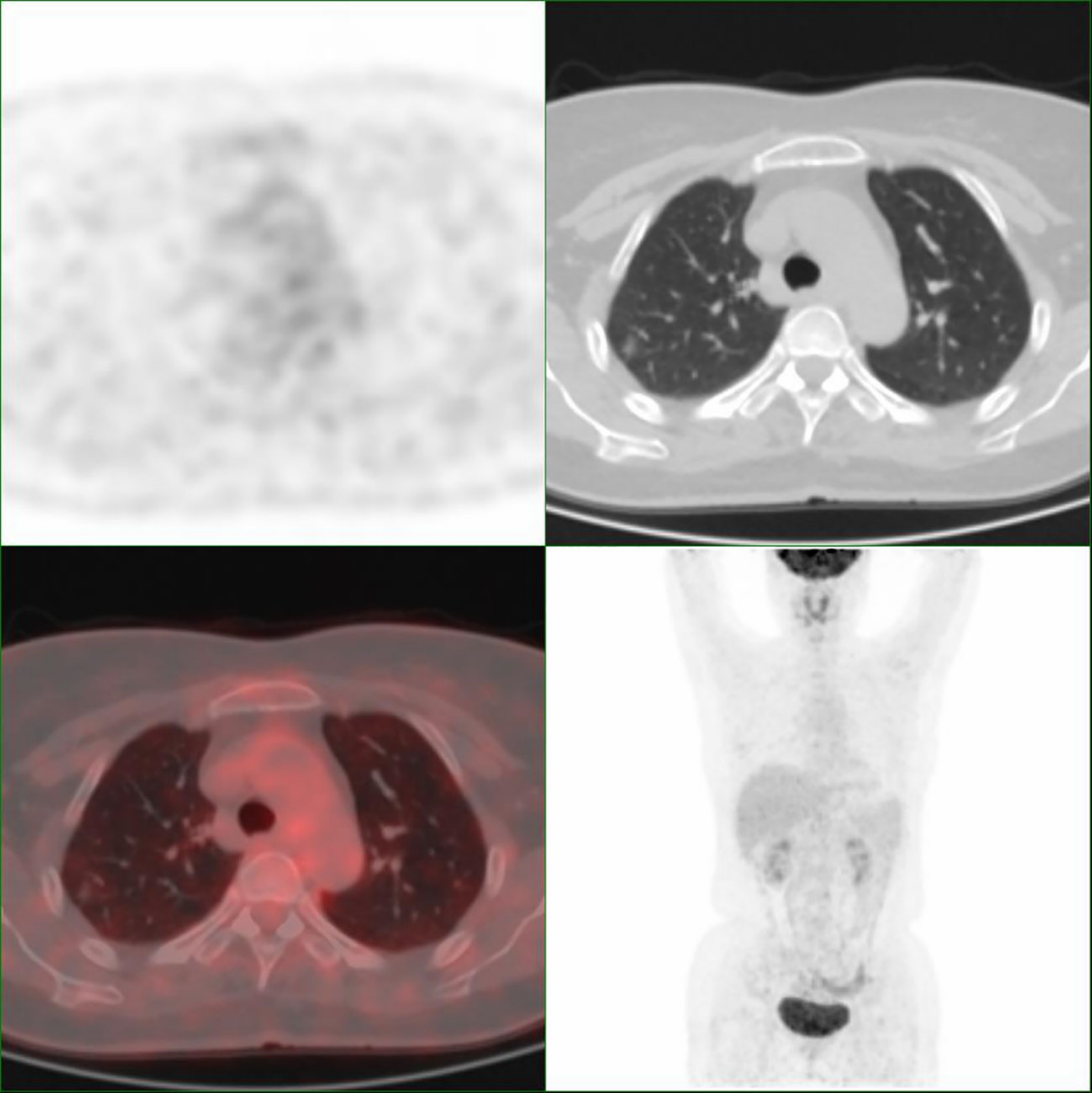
Representative images of Patient 4 with adenocarcinoma *in situ*. The nodule has an SUVmax of 0.9.
